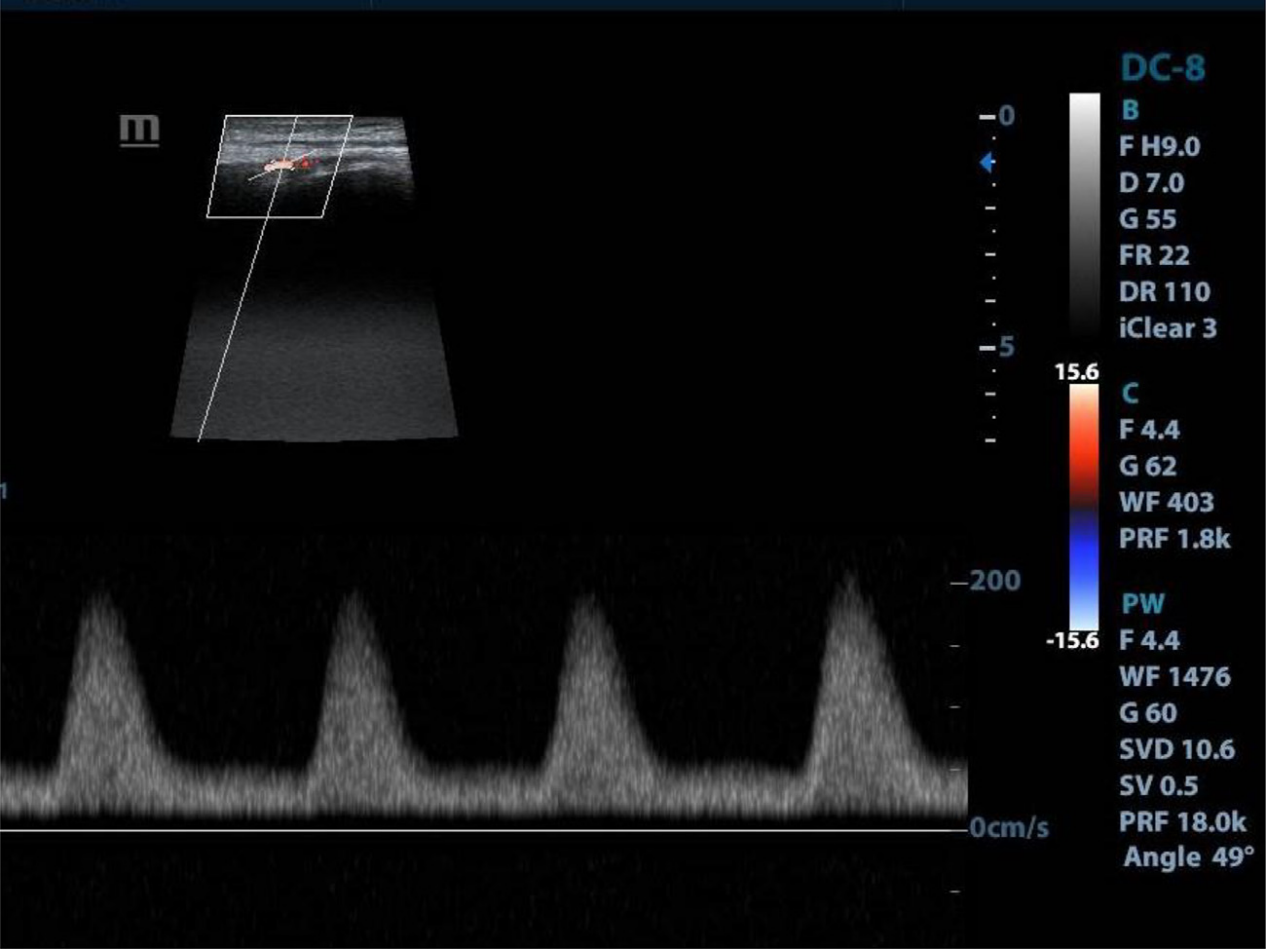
Doppler ultrasound of the left lower limb: There appears to be a monophasic waveform in the left superficial femoral artery.
